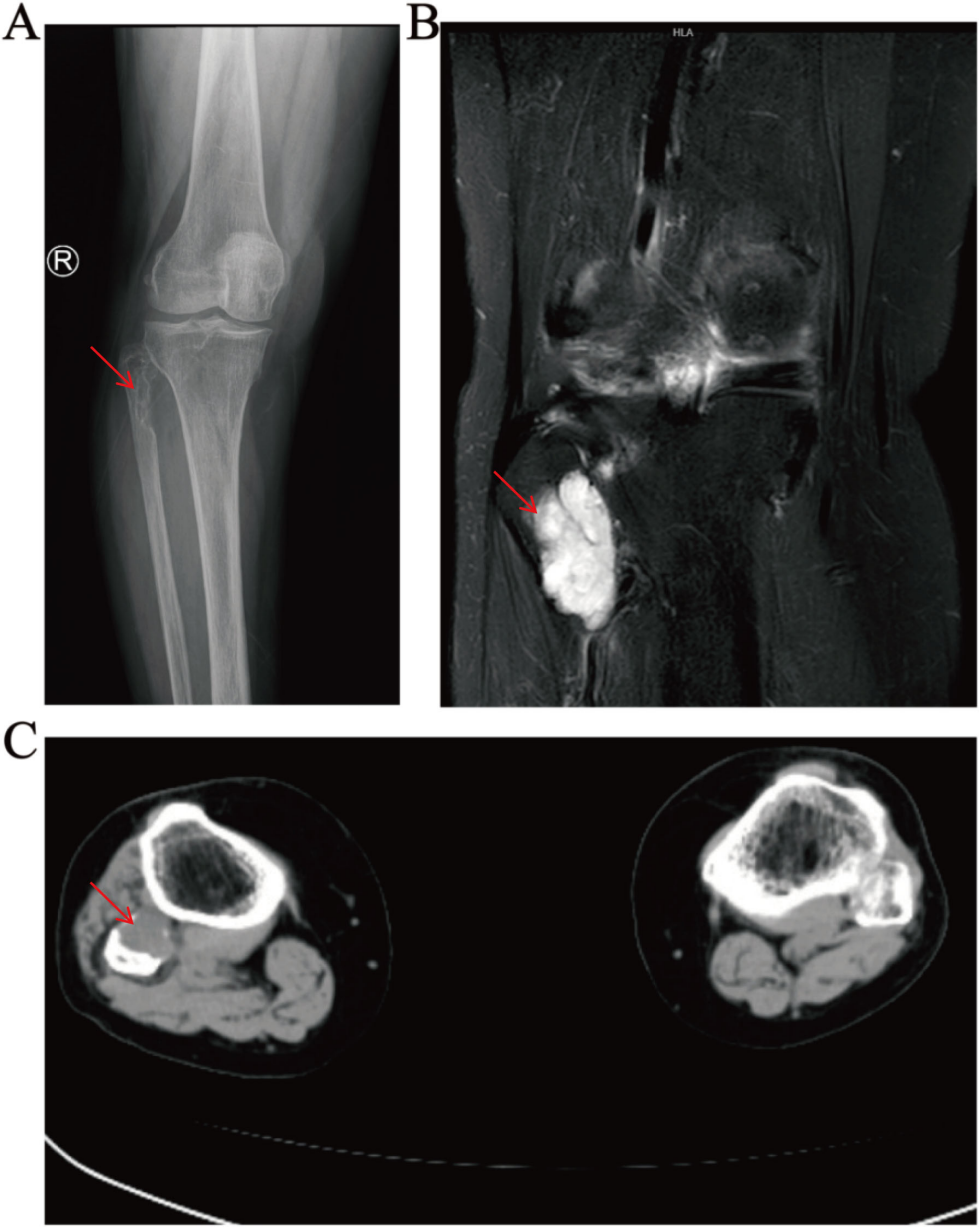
Preoperative imaging of the patient. (A) X-ray: bone defect in the proximal part of the fibula with uneven bone density, (B) enhanced MRI: tibiofibular interosseous soft-tissue mass, (C) CT: fibular head and tibiofibular interosseous soft-tissue mass.
